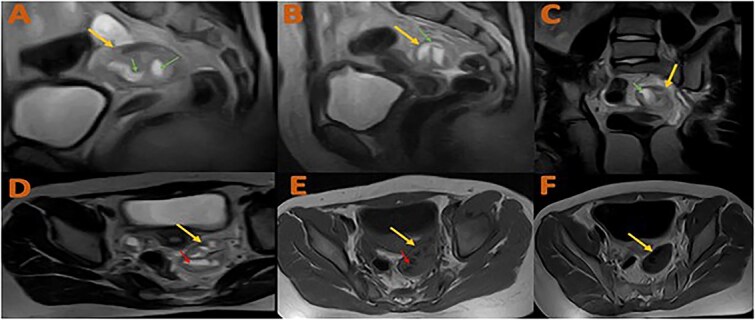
Contrast-enhanced MRI of the pelvis. (A and B) sagittal T2WI. (C) Coronal T2WI; (D) axial T2WI; (E and F) axial T1 TWI pre- and post-contrast administration. Shows well-defined heterogenous mass involving the left adnexa between the uterus and left ovary ( arrows), which contains dilated fluid-filled T2 hyperintense fallopian tube with multiple fluid levels (small arrows). The mass shows no enhancement post-contrast administration.
